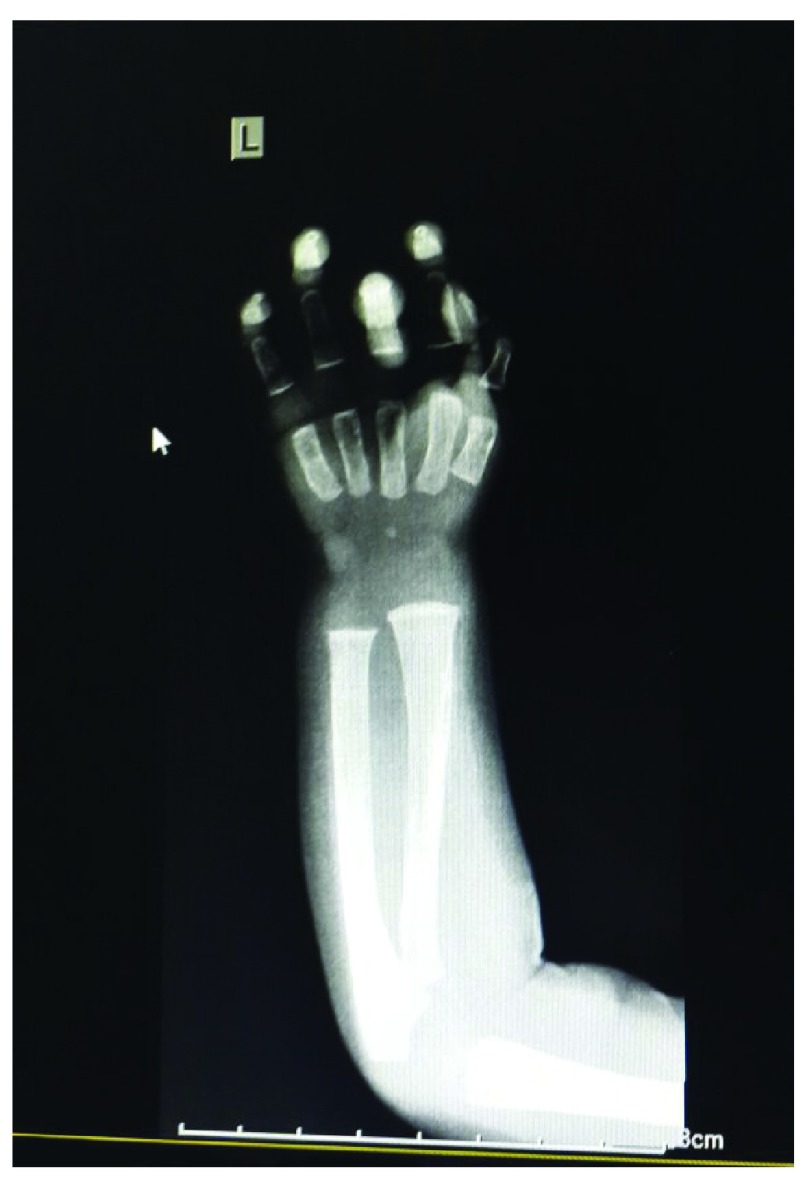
X-ray of left hand showing ectrodactyly.
